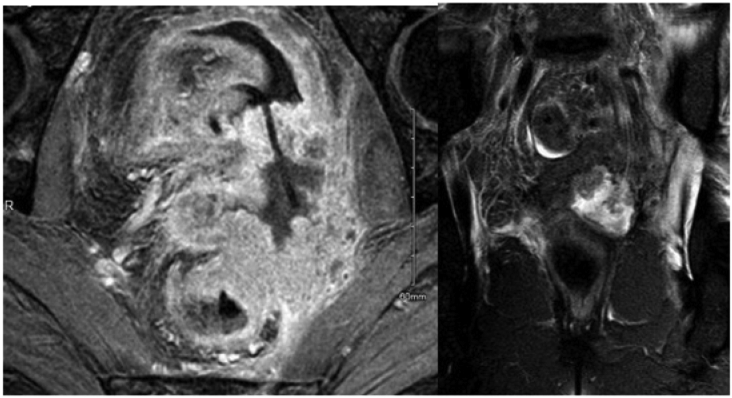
Axial and coronal MRI sequences demonstrating locally advanced parametrial tumour invading the urinary bladder wall, rectum and vagina. Complete encasement of the distal left ureter with large uretero-vaginal fistula. Left ureteric stent in situ.
